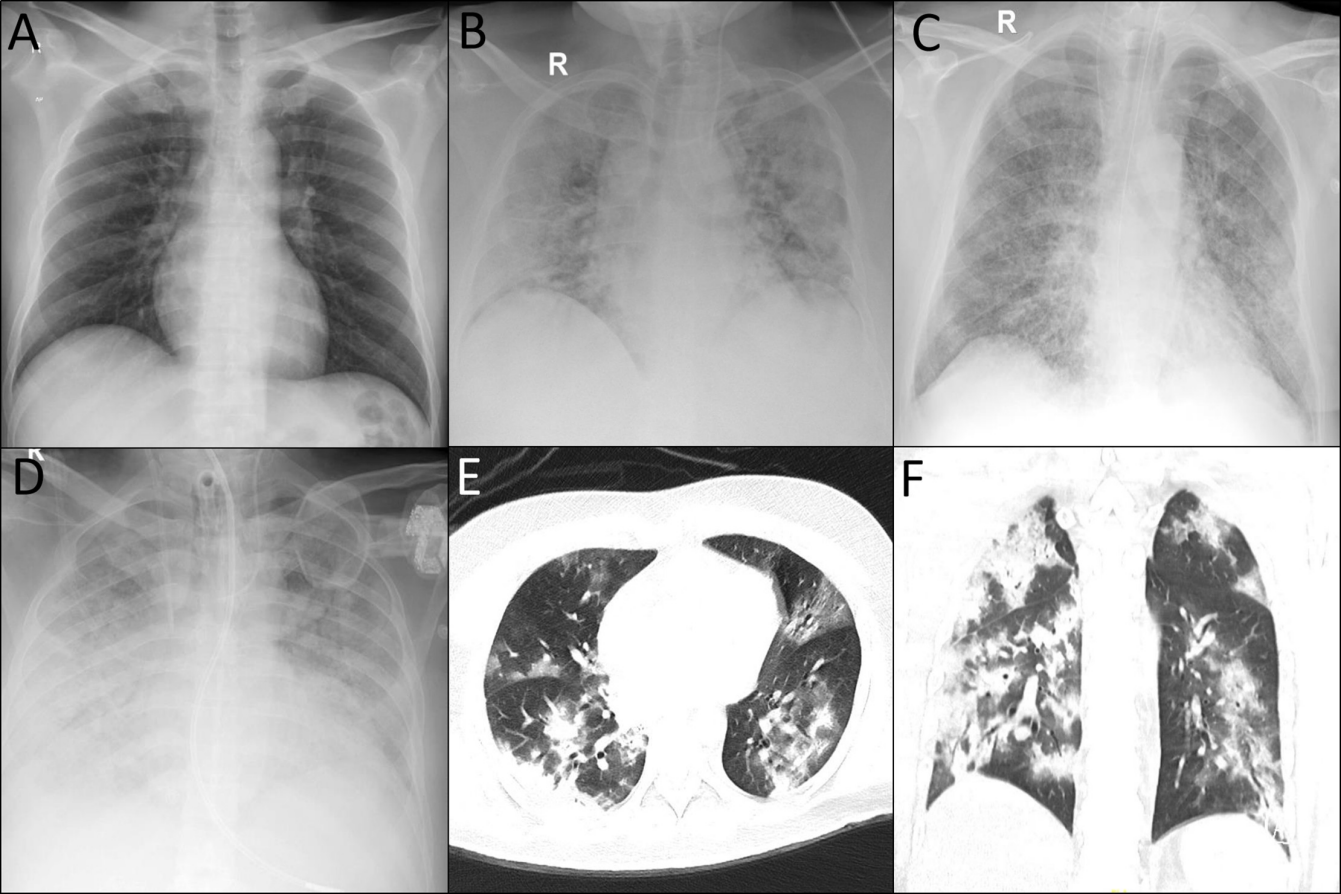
COVID-19 patients chest imaging. A. Normal chest radiography of a patient with mild to moderate COVID-19. B. Diffuse bilateral airspace opacification with patches of consolidation in an ICU survivor. C. Diffuse bilateral airspace opacification with consolidation patches in an ICU death. D. Diffuse bilateral airspace opacification with near total white out of both lung fields in an ICU death. E and F. High Resolution CT chest showing multifocal large patchy areas of ground glass opacification mixed with dense consolidations in an ICU death.
